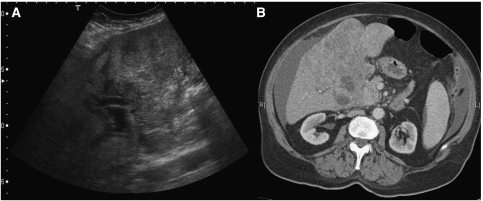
True positive. Stage IV breast cancer patient. Ultrasound liver shows irregular lesions within the liver (A), subsequent CT (B) confirms liver metastases, ascites also demonstrated.
